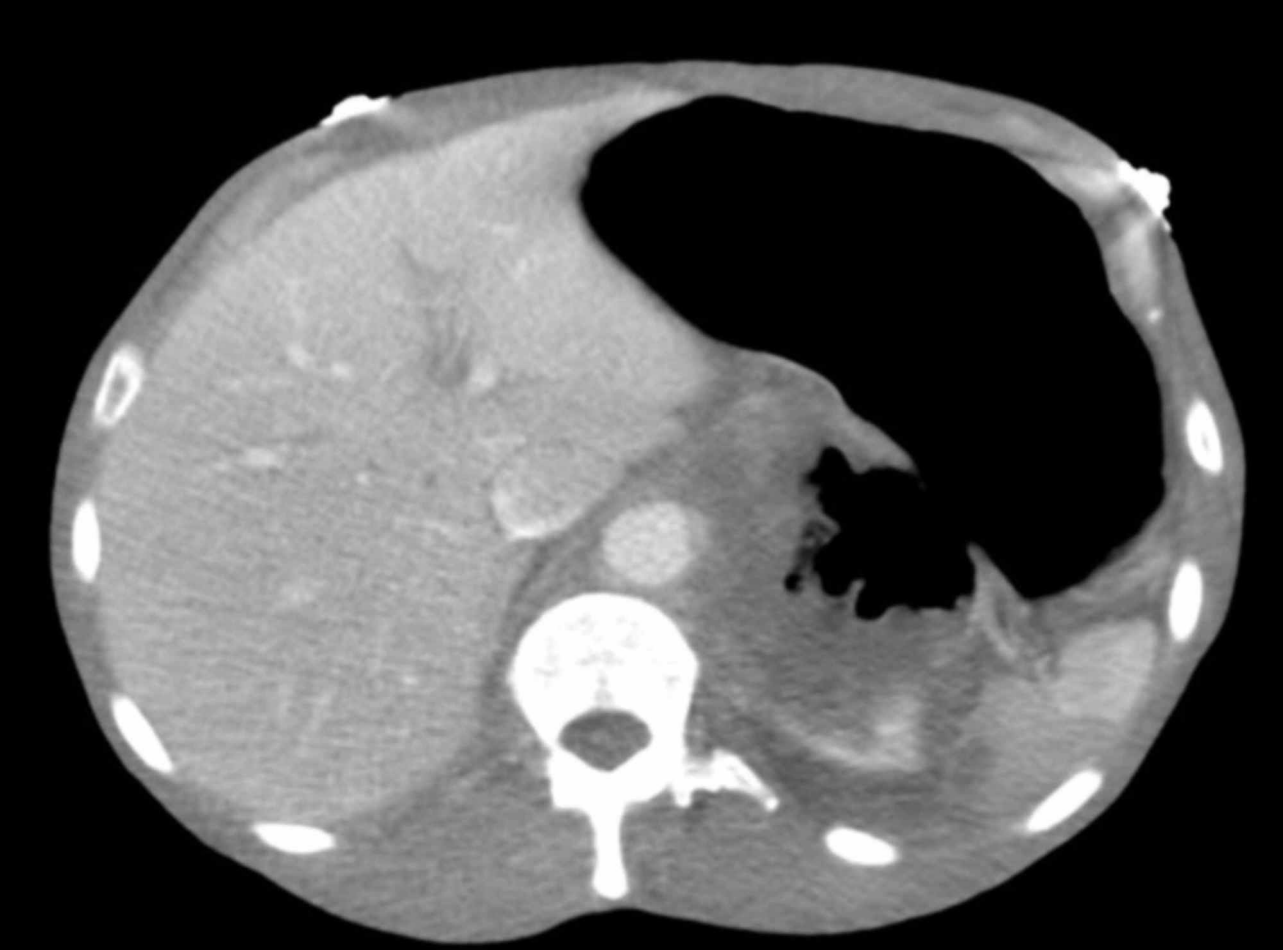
Pre-contrast CT scan of the abdomen with stomach dilation, increase in renal mass, and presence of fistula between stomach and kidney mass.
